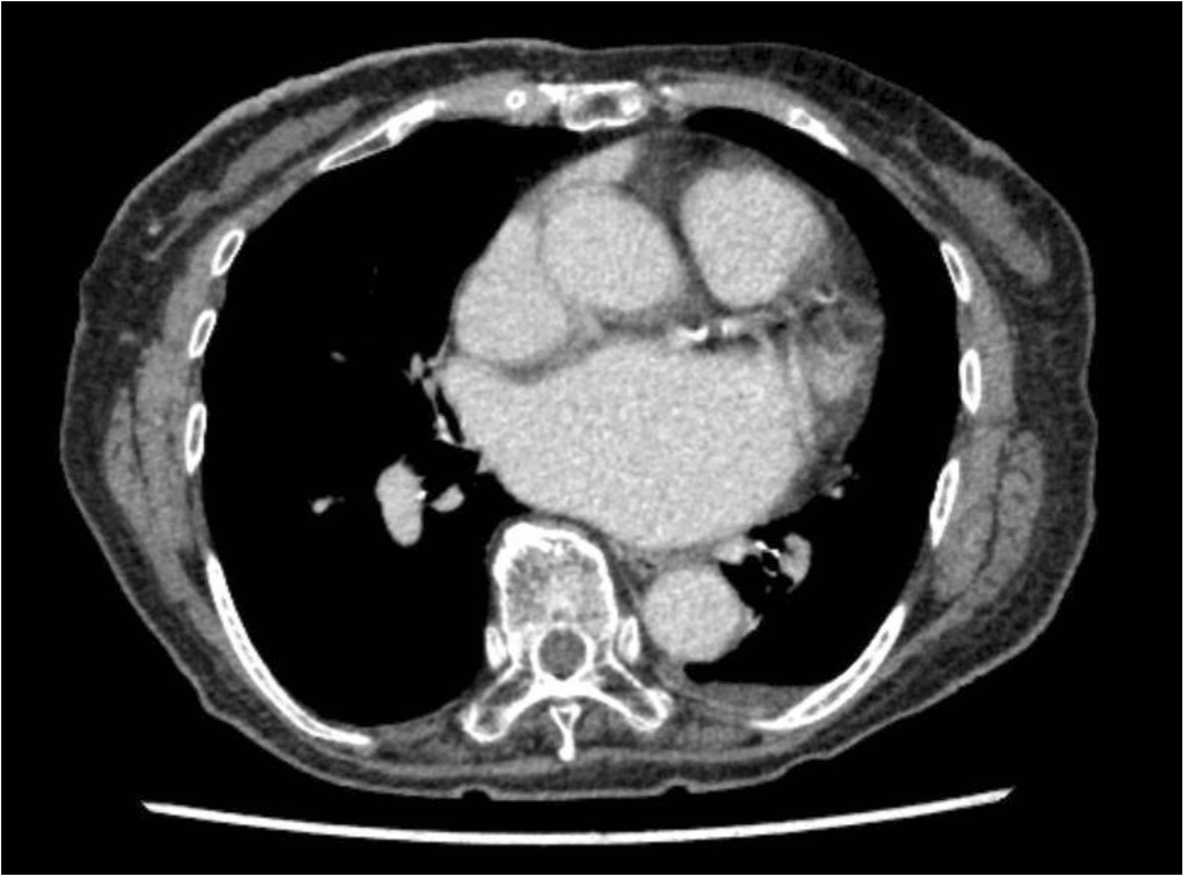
Computed tomography. The tumor was only on the surface of the breast without any underlying tumor
